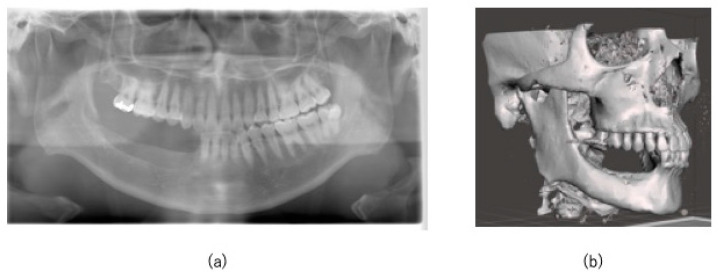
Postoperative 3-year follow-up imaging. (a,b) OPG and CT at the 3-year follow-up shows no recurrence and no sensory abnormalities in the inferior alveolar nerve.
